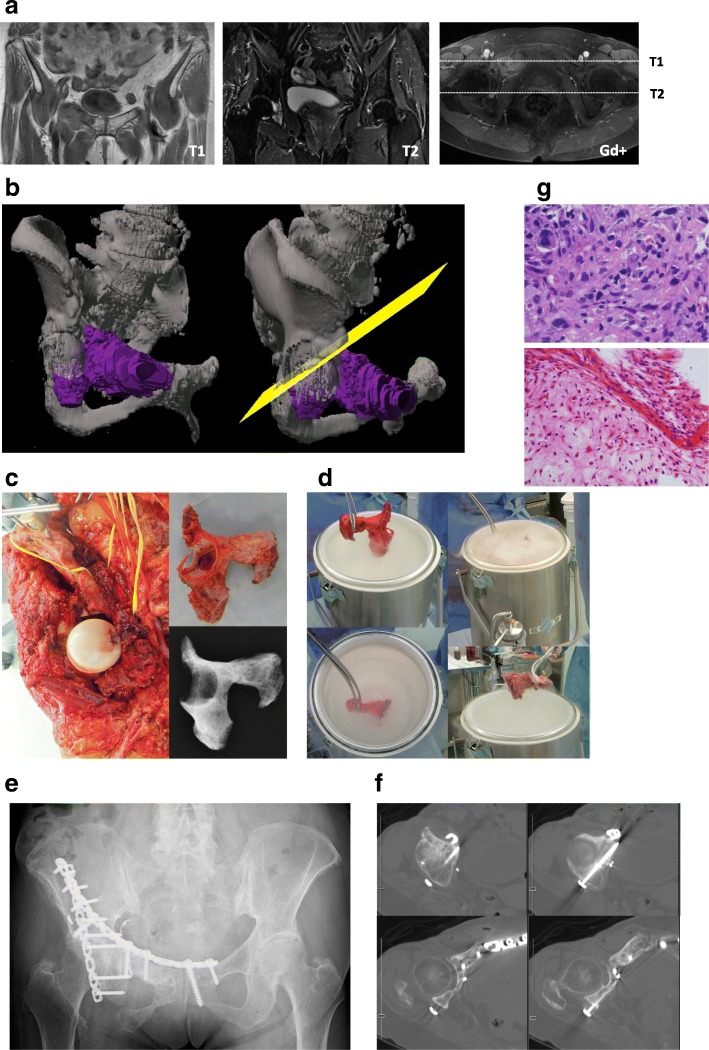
Preoperative and postoperative assessments in case 2: A 71-year-old woman with dedifferentiated chondrosarcoma of the pelvis (PII-III). a) Preoperative magnetic resonance imaging. b Preoperative plan involving the use of a computer navigation system. The osteotomy line was planned as indicated by the yellow surface. c After osteotomy d Procedure of freezing in liquid nitrogen. e Radiograph obtained at 33 months after reconstruction. f Computed tomography at 33 months after reconstruction. Bone union is observed, and osteoarthritis is not very prominent. g Pathological findings at biopsy (upper panel) and post-neoadjuvant chemotherapy (lower panel). After neoadjuvant chemotherapy, a necrotic change was observed
